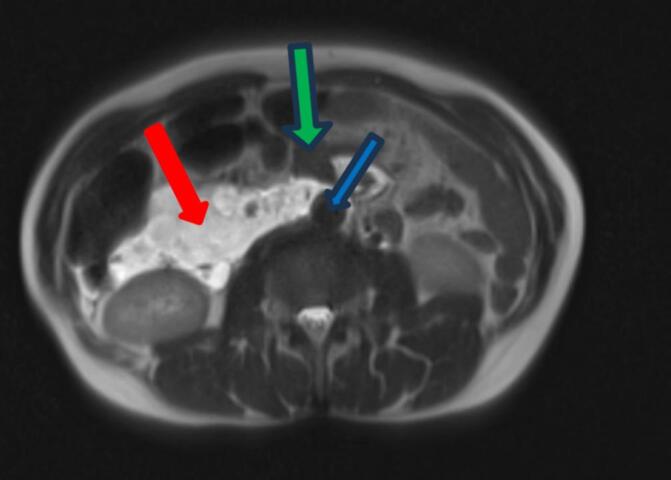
T2-weighted axial section at the top of the renal pedicle on pelvic MRI. The extension of the mass (red arrow) is in intimate contact with the aorta (blue arrow) as well as the head of the pancreas (green arrow). (For interpretation of the references to colour in this figure legend, the reader is referred to the web version of this article.)
